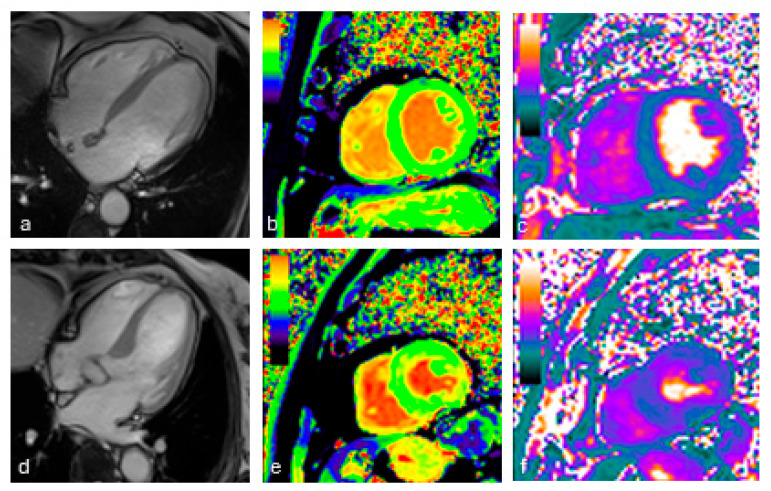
Cardiac MRI images from two COVID-19 survivors: a 62-year-old man without hypertension (a–c) and an 87-year-old woman with hypertension (d–f). For the patient without hypertension, we depict a steady-state free-precession four-chamber view (a), with a native T1 of 1190 ms, resulting in a normal ECV of 22.0% (b), and a T2 of 39 ms (c). For the patient with hypertension, we demonstrate a steady-state free-precession four-chamber view (d), with a native T1 of 1320 ms, an elevated ECV of 34.9% (e), and a T2 of 47 ms (f).
